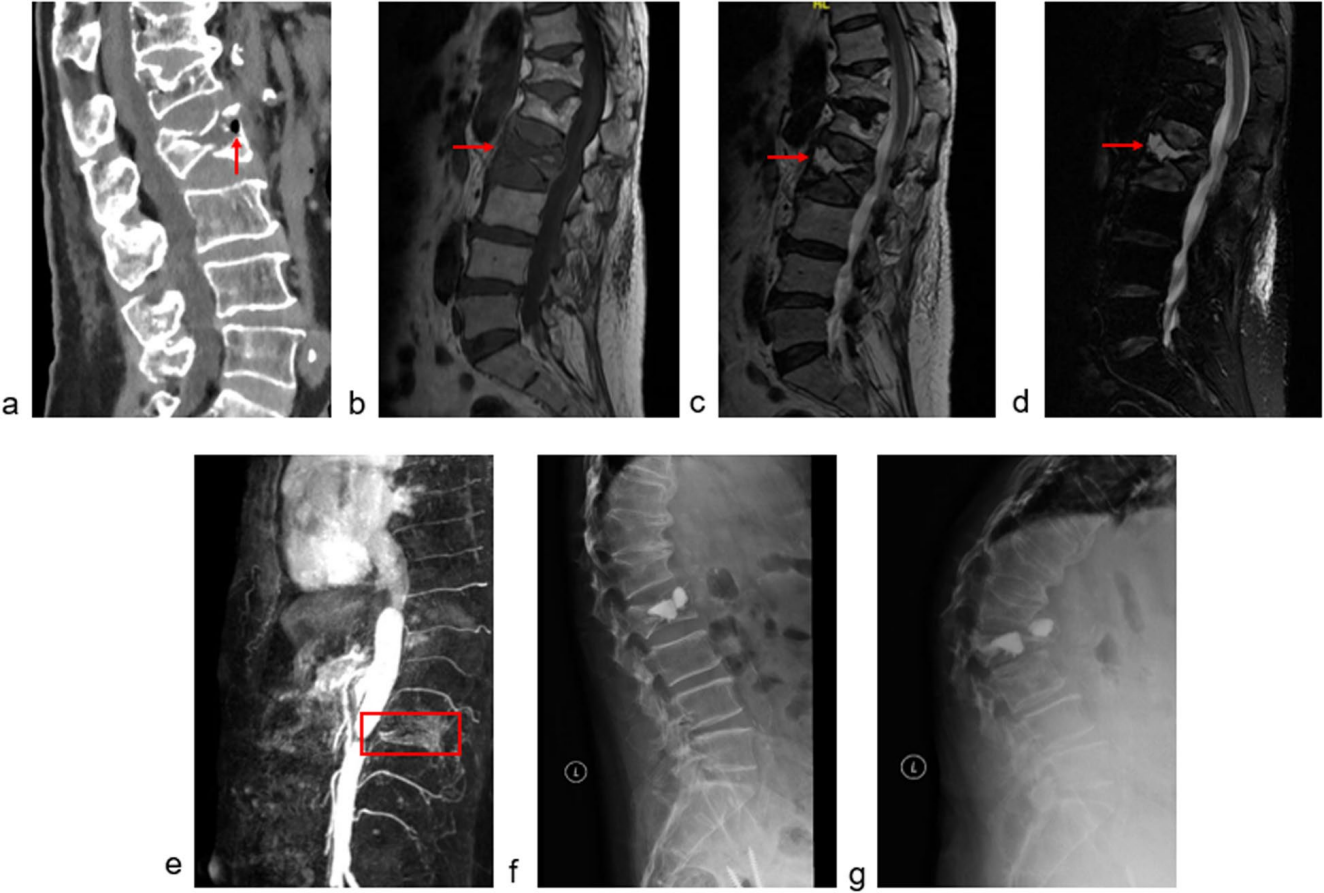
An eighty-two-year-old female suffered from severe back pain for about 17 days (VAS 9) after left heavy object. CT showed an L2 compression fracture with intravertebral cleft formation (a) and MRI indicated an area of hypointensity on T1 and hyperintensity on T2 and T2-fat suppression images (b, c, d, red arrow). MRA revealed that both sides of the L2 segmental arteries were occluded (e). Unilateral vertebroplasty was performed for the patients (f). The augmented vertebra collapsed and the cement was displaced half a year after the procedure (g)
